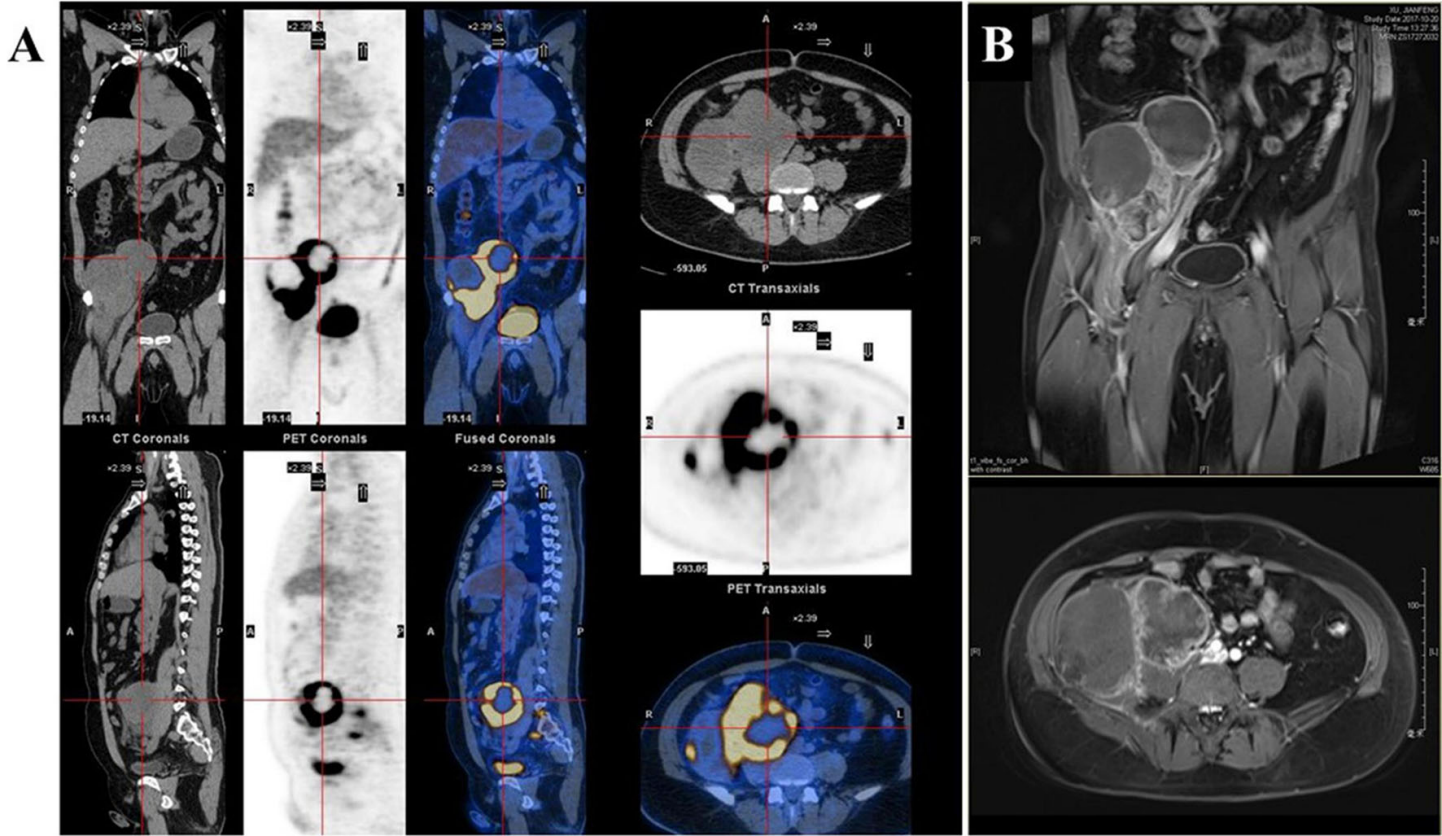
(A) Positron emission tomography/computed tomography (PET/CT). (B) Magnetic resonance imaging (MRI) demonstrated a malignant tumor involving both the right psoas major muscle and anterior iliac fossa.
